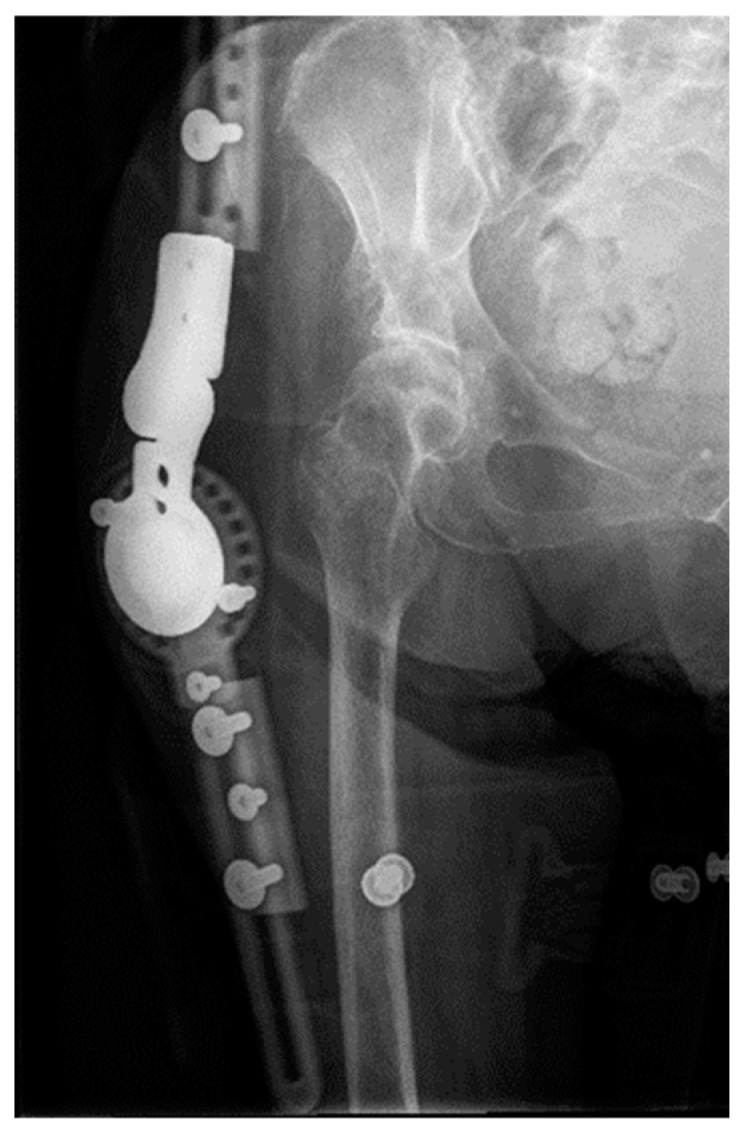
Control radiography at 20 months after the first intervention.
